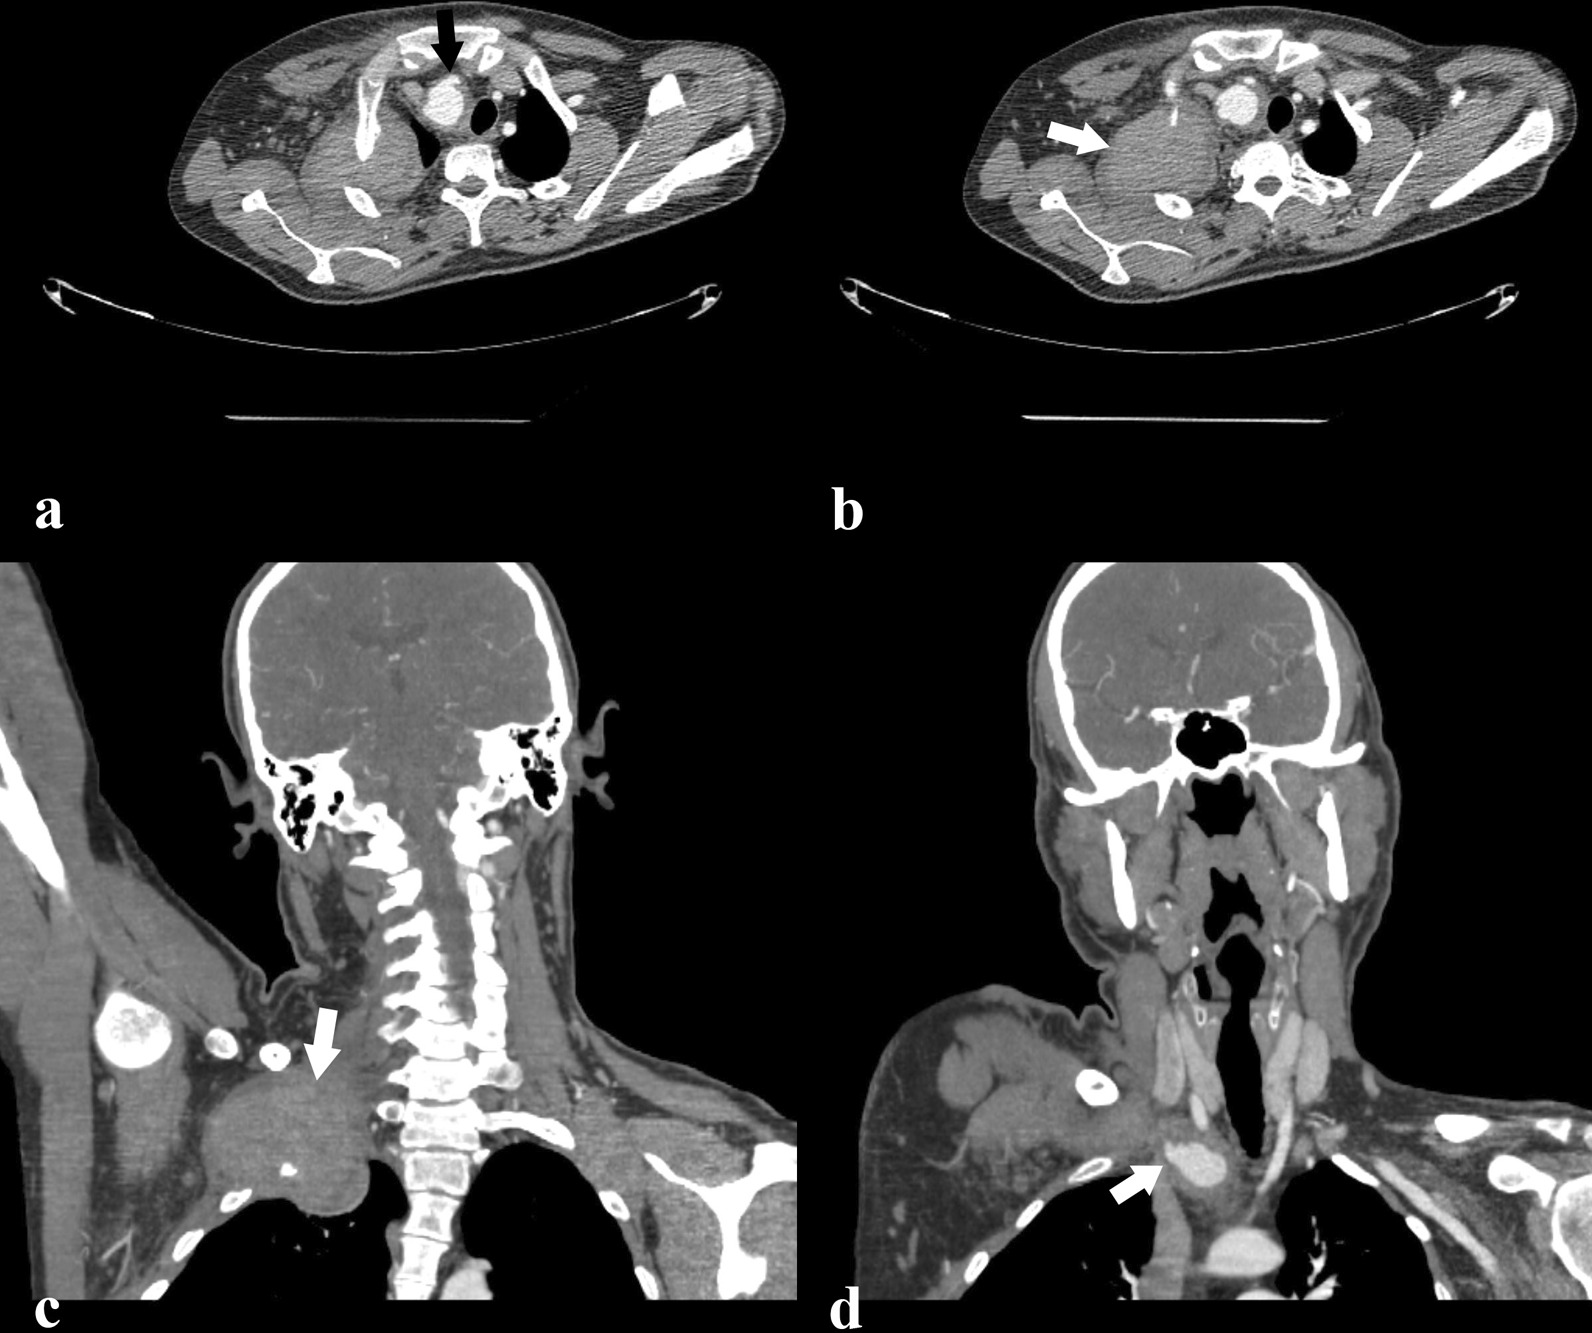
Preoperative contrast-enhanced computed tomography angiogram (CTA). a Axial image: the pseudoaneurysm of the innominate artery causing pressure effect on the trachea (black arrow). b Axial image: the ruptured pseudoaneurysm hematoma (white arrow). c Coronal reconstruction: a large hematoma is observed in the right shoulder extending to the extrapleural area in the apex of the right thoracic cavity (white arrow). d Coronal reconstruction: innominate artery pseudoaneurysm is observed (white arrow). It must be noted that the right subclavian artery is occulted
